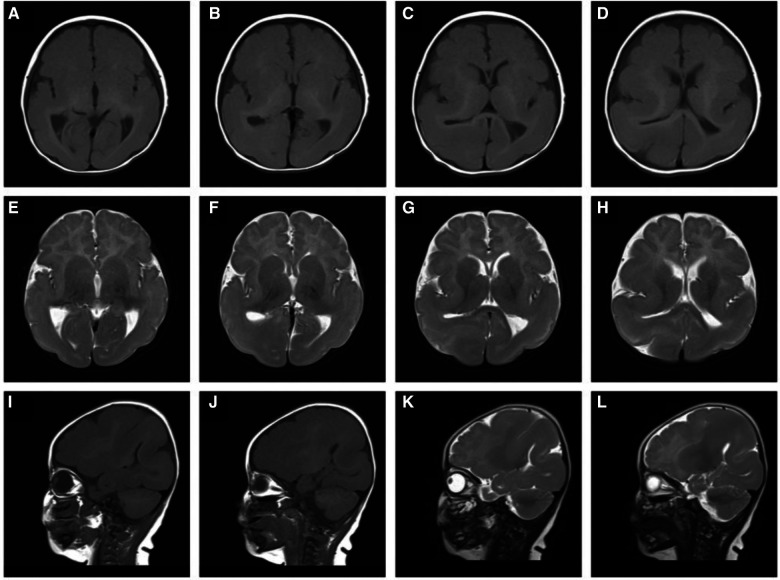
T1-weighted sagittal (A–D), T2-weighted sagittal (E–H), T1-weighted axial (I, J), T2-weighted axial (K, L) magnetic resonance imaging: showing abnormal changes bilaterally in the temporo–parietal occipital gyri and sulci, pachygyria and lissencephaly.
